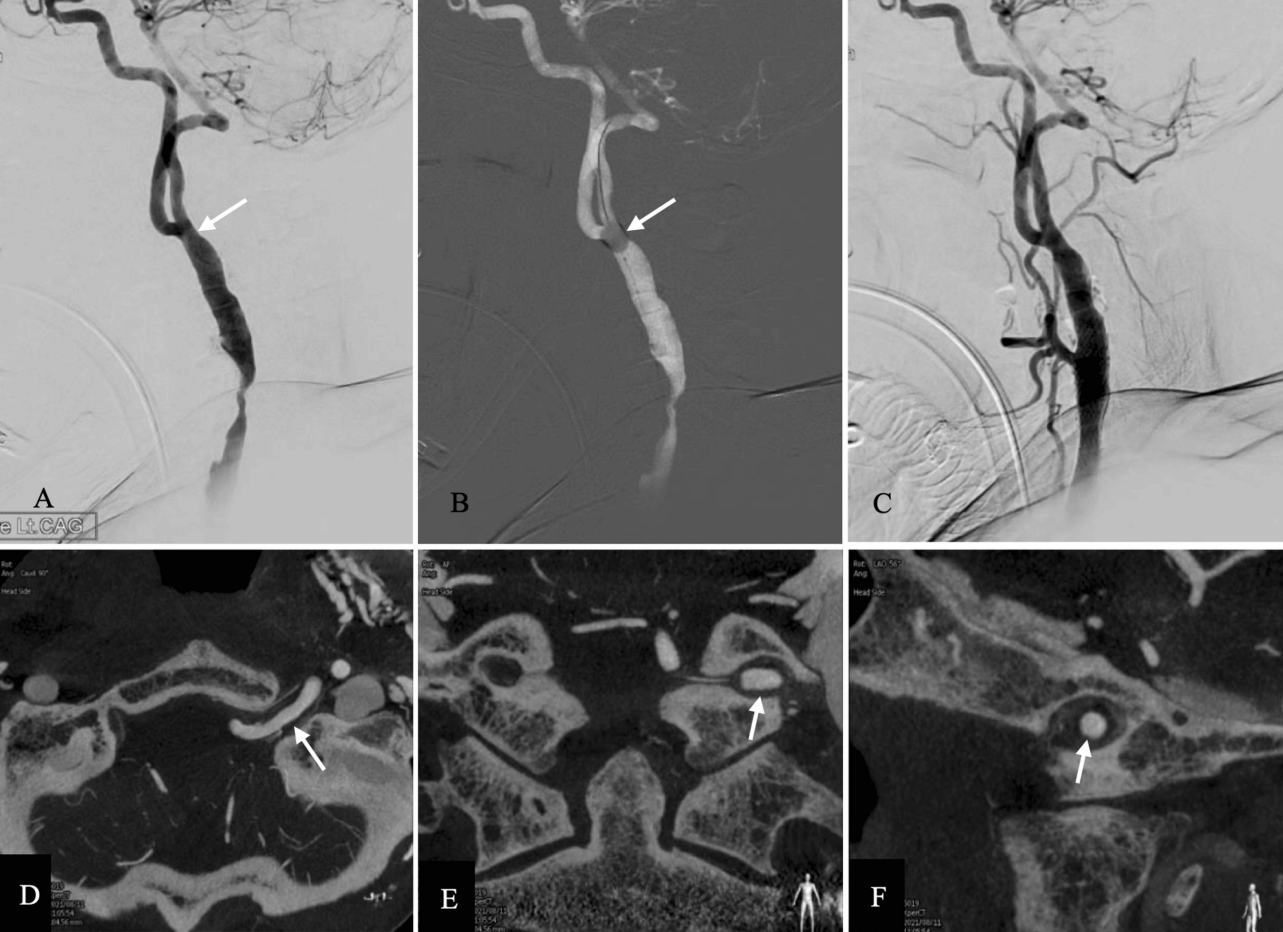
Treatment and cone beam CT of the PPHA. (A) Preoperative left CCA injection showing CCA stenosis. The caliber of the distal CCA is too large to occlude with a distal balloon protection device. Note the narrow segment at the bifurcation (white arrow). (B) Intraoperative roadmap. The distal balloon (white arrow) protection device is located at the ICA and PPHA bifurcation, where its diameter is small enough for the distal balloon protection devices. (C) Postoperative injection showing successful stenting. Cone beam CT revealing left PPHA (white arrows) running through the hypoglossal canal: (D) axial, (E) coronal, and (F) sagittal CT: computed tomography; PPHA: persistent primitive hypoglossal artery; CCA: common carotid artery
